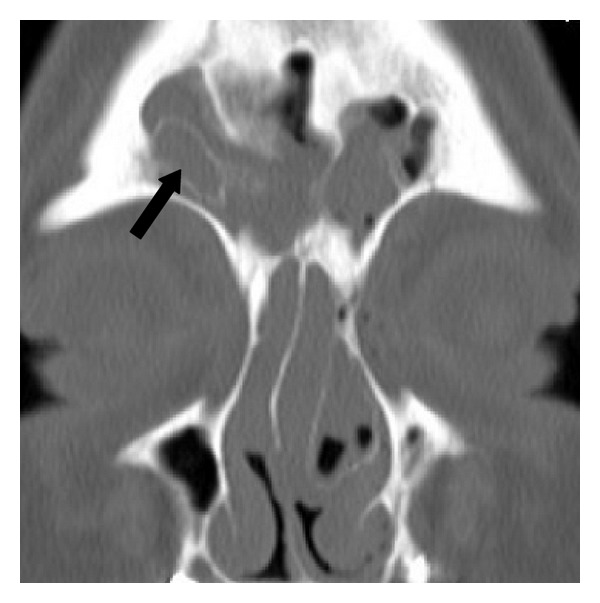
Type IV frontal cell. A coronal CT scan showing a right-sided type IV frontal cell (arrow) lying completely within the frontal sinus.
